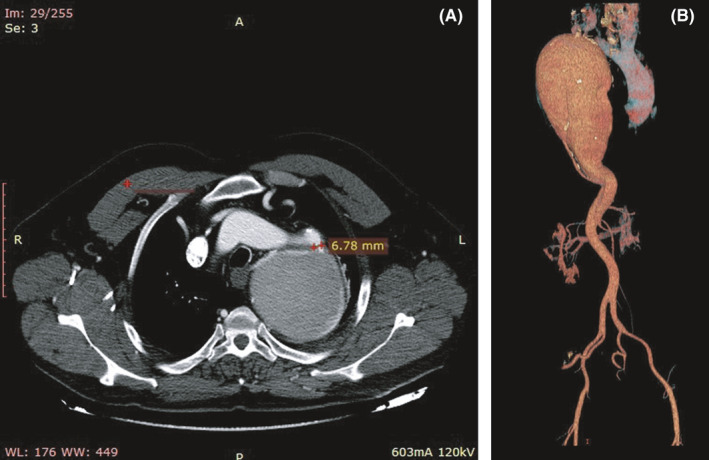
(A) Descending aorta presented extremely dilated, with a transverse diameter approximating ten centimeters and with a coarcted portion proximally with a diameter of less than 7 mm (markers). (B) Angio CT reconstruction images showing the giant aneurysm
